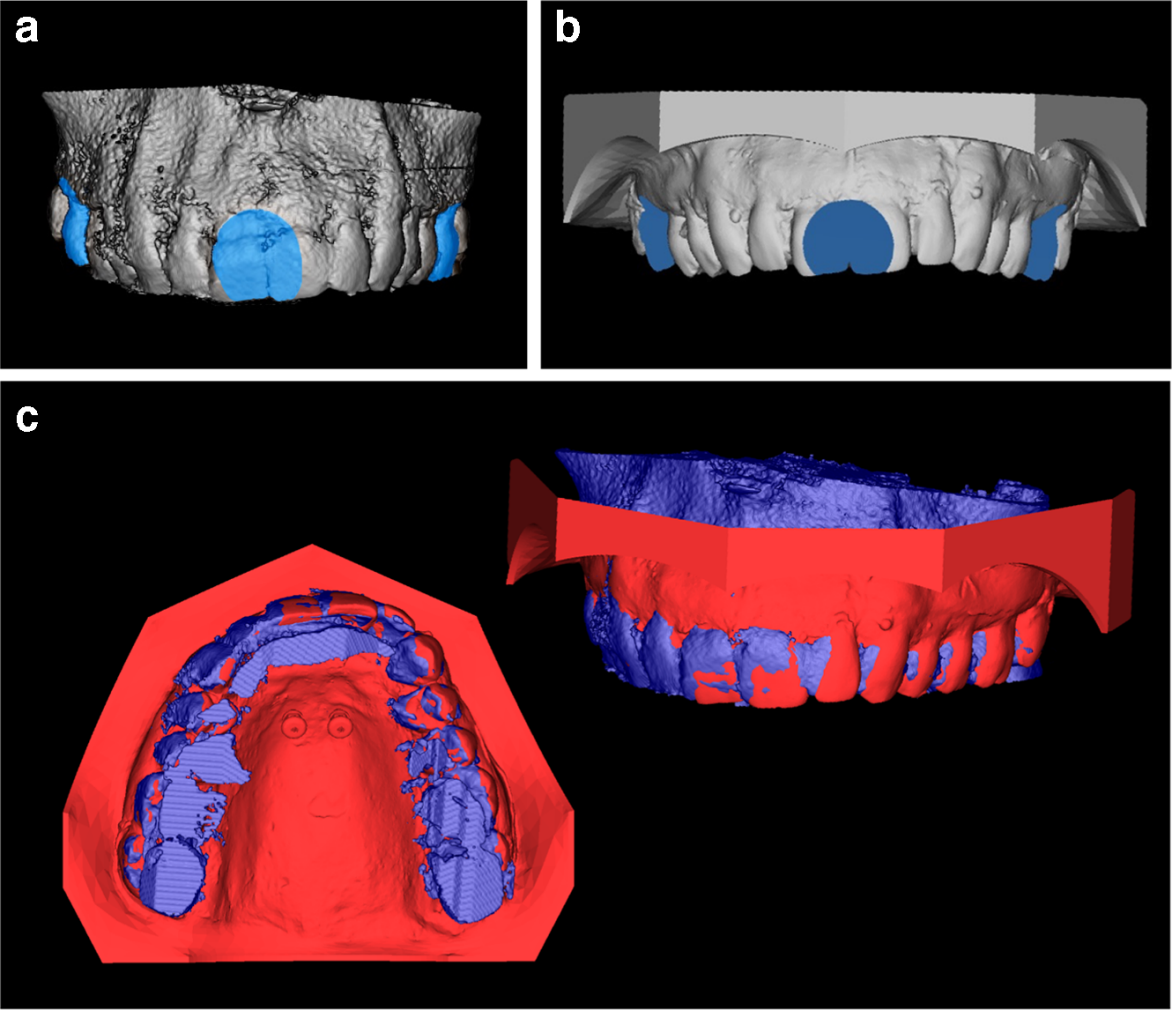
a At least three matching references spread over the dental arch were chosen. b Additionally, the corresponding matching references were marked on the cast model. c Matching of the radiographic file and cast model data using coDiagnostiXTM software
